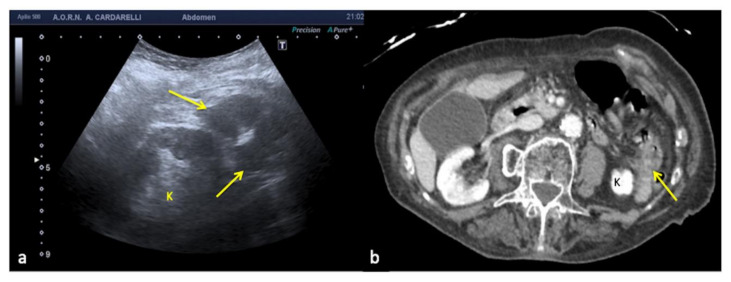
Longitudinal US left flank scan (a) shows at the lower pole of the kidney (K) a hypoechoic area which may be misinterpreted as retroperitoneal free fluid (arrows) in a 22-year-old man investigated for trauma. On CT scan (b) it appears to be a spastic intestinal loop (arrow).
